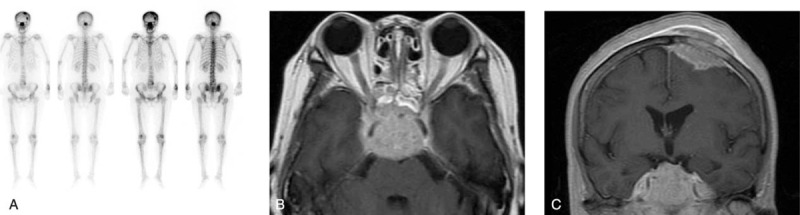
SPECT and MRI revealed that the pituitary mass recurred with multiple bone metastasis and intracranial metastasis after surgery. (A) SPECT performed 1 month after surgery reveals the tumor metastasis in the skull, skull base, 3rd thoracic vertebra, and the right sacroiliac joint. MRI performed 3 months after surgery reveals the pituitary mass (B) and left frontoparietal mass (C) that recurred. MRI = magnetic resonance imaging, SPECT = single-photon emission computed tomography.
